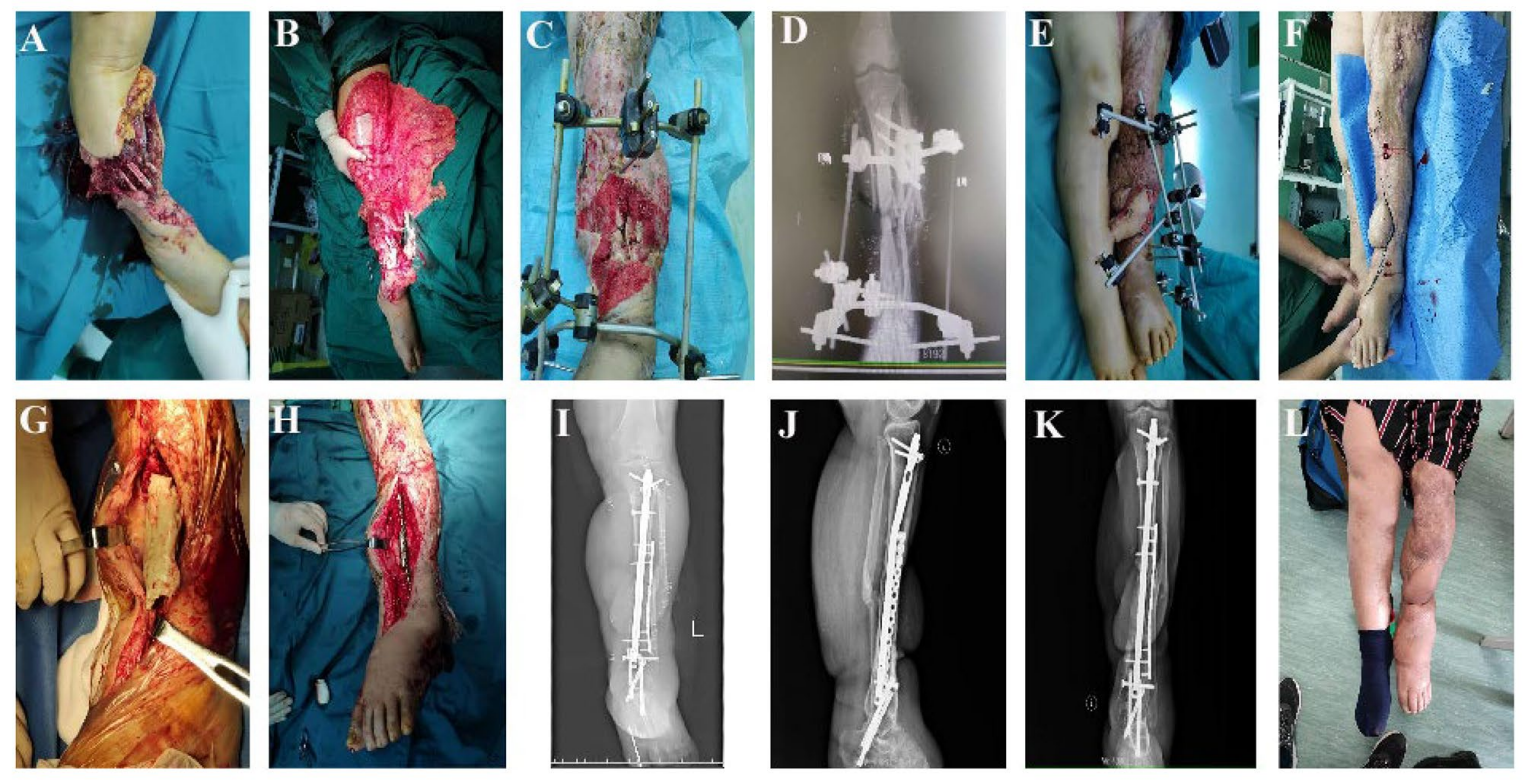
A 27-year-old female patient (case 6) with Gustilo IIIB open tibial fracture. (A, B) Photograph of wound and bone defects before operation. (C, D) After debridement, external fixation was performed. (E) the defect was filled with bone cement spacer, external fixation and soft tissue repair with Crossover posterior tibial artery perforator flap were performed. (F-I) 12 weeks later, cancellous bone grafting was performed (J-L) Radiograph and photograph of 12 months post implantation operation
